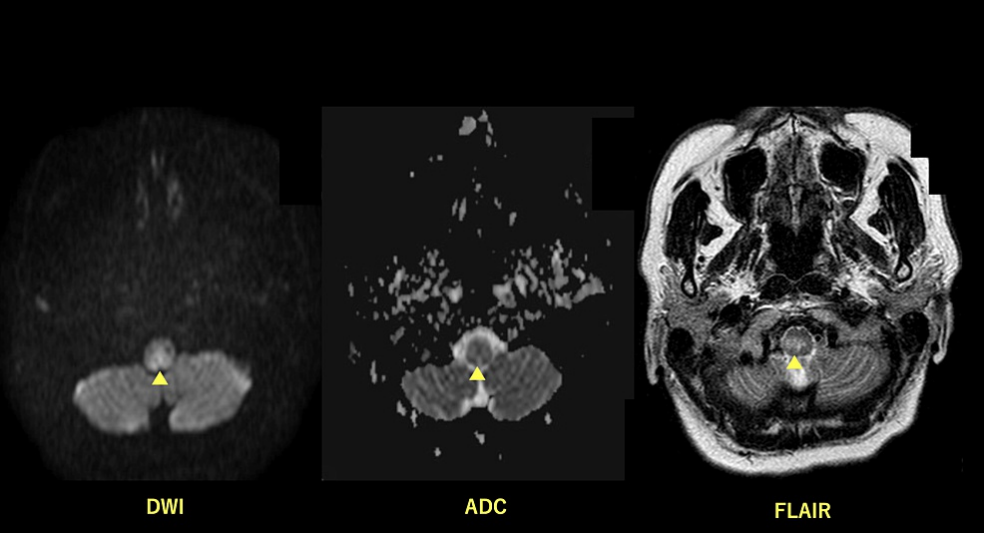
Brain magnetic resonance imaging scan diffusion-coordinated image showing a high-intensity area in the medulla oblongata. No ADC reduction was observed, and T2WI and FLAIR showed high intensity in the same area. DWI: diffusion-weighted image; ADC: apparent diffusion coefficient; T2WI: T2-weighted imaging; FLAIR: fluid-attenuated inversion recovery
